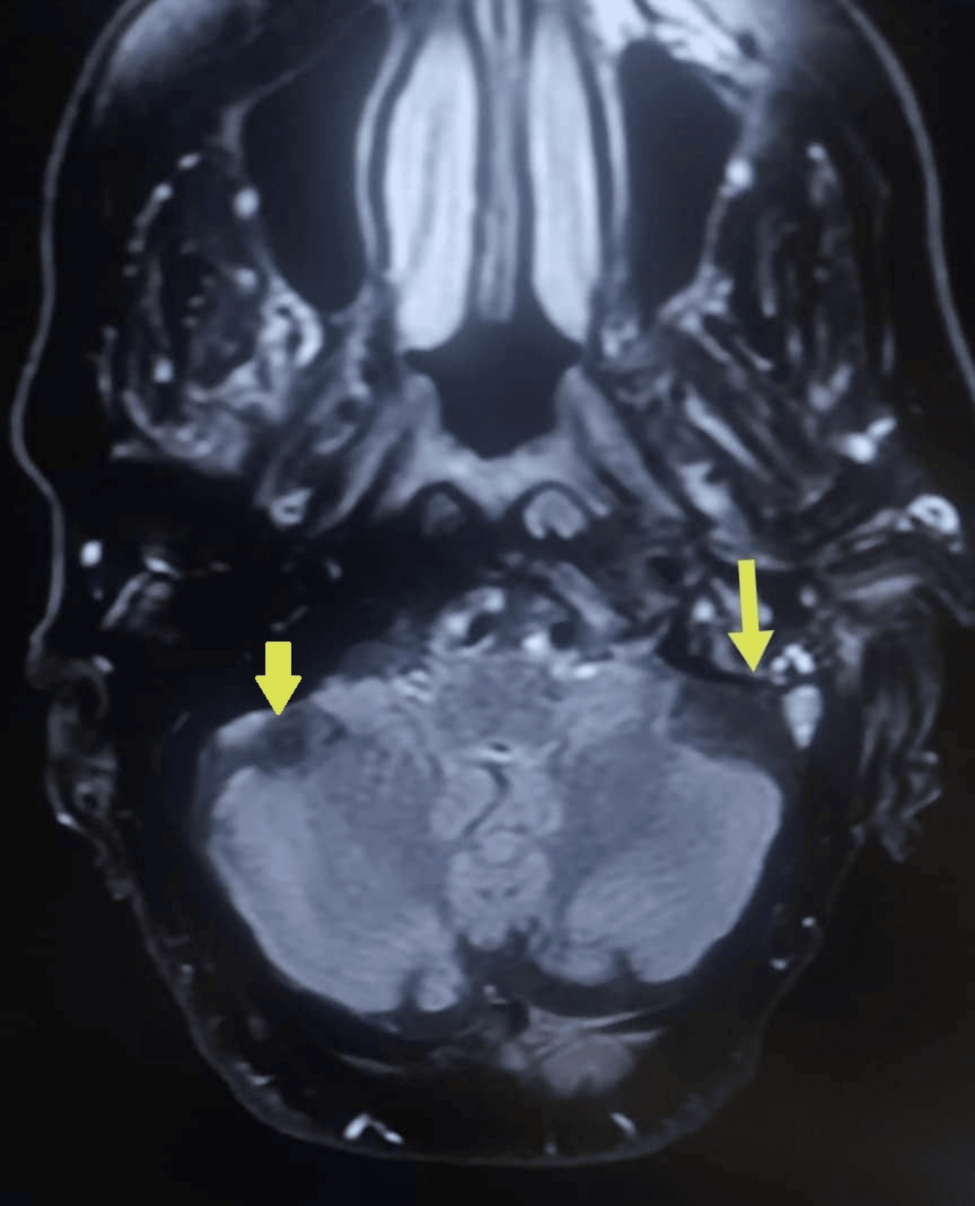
Magnetic resonance fluid-attenuated inversion recovery (FLAIR) sequence demonstrating increased signal intensity at the junction of the sigmoid sinus and internal jugular vein, suggestive of slow flow or thrombosis (Yellow Arrows).
